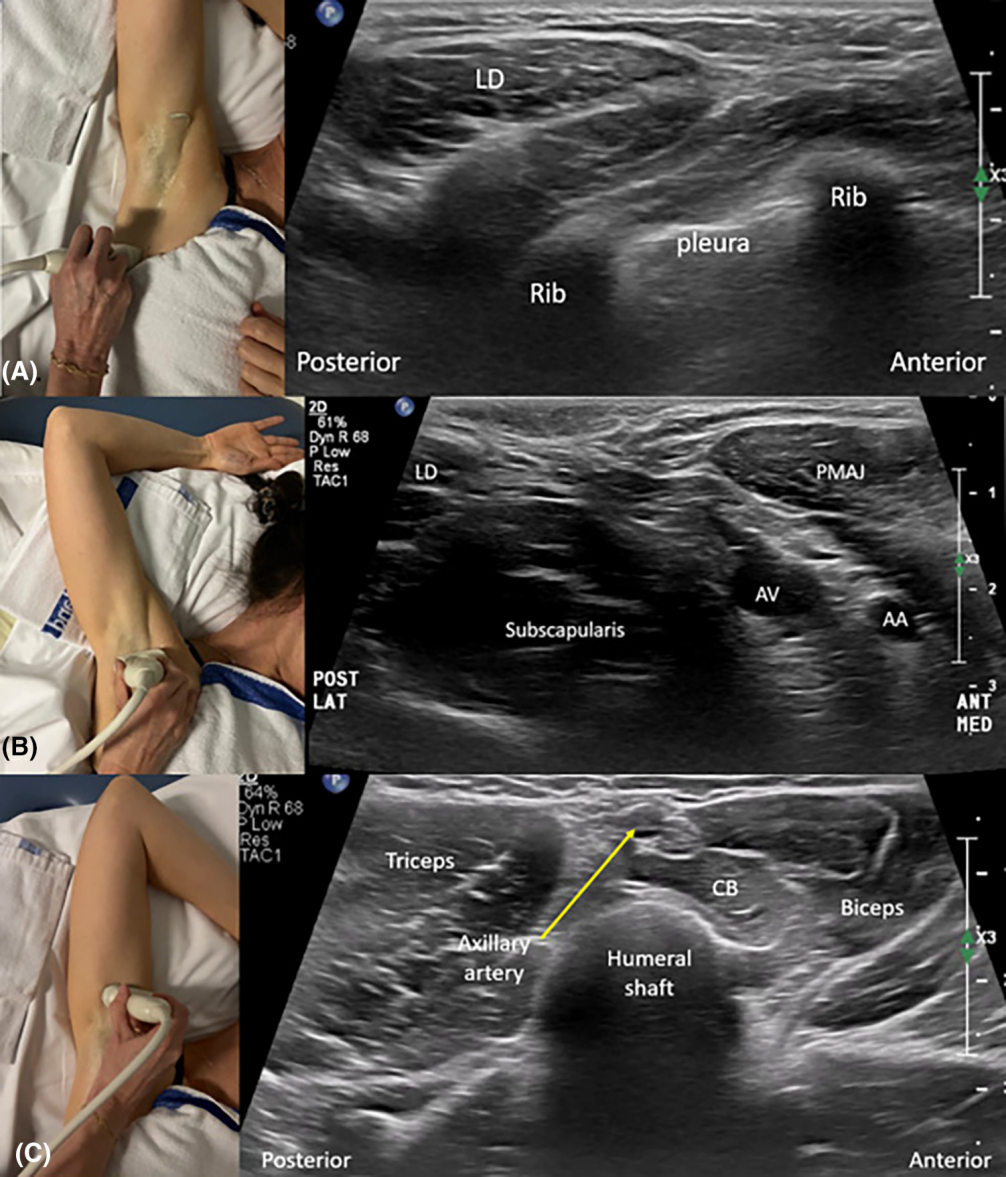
Short‐axis static sonographic image of Level I of the axilla. AA, axillary artery; AV, axillary vein; LD, latissimus dorsi; PMAJ, pectoralis major.
